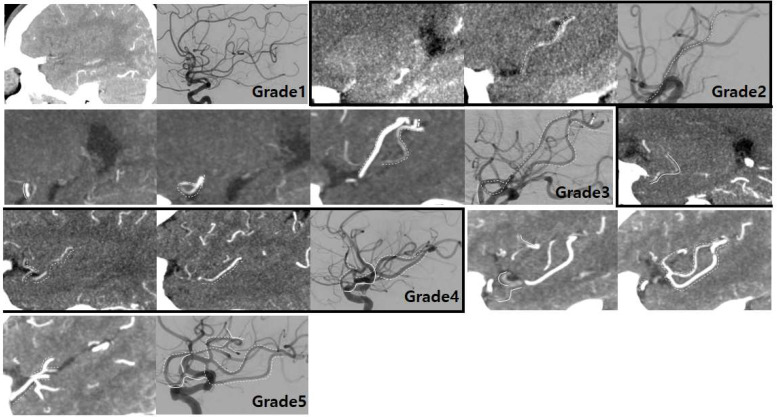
CTA grading of distal-to-thrombus segment in correspondence to the reference standard of final DSA findings. CTA showed no MCA course at all (grade 1), only a small portion of the inferior division of MCA (grade 2), only a small portion of the superior and inferior division of MCA (grade 3), almost all of the inferior division and small portion of the superior division of MCA (grade 4), and all of the inferior division and superior division of MCA (grade 5), respectively. The arterial courses on CTA were matched with those on DSA as indicated by solid (MCA proximal portion) and dotted lines (MCA distal portions). CTA, computed tomography angiography; DSA, digital subtraction angiography; MCA, middle cerebral artery.
